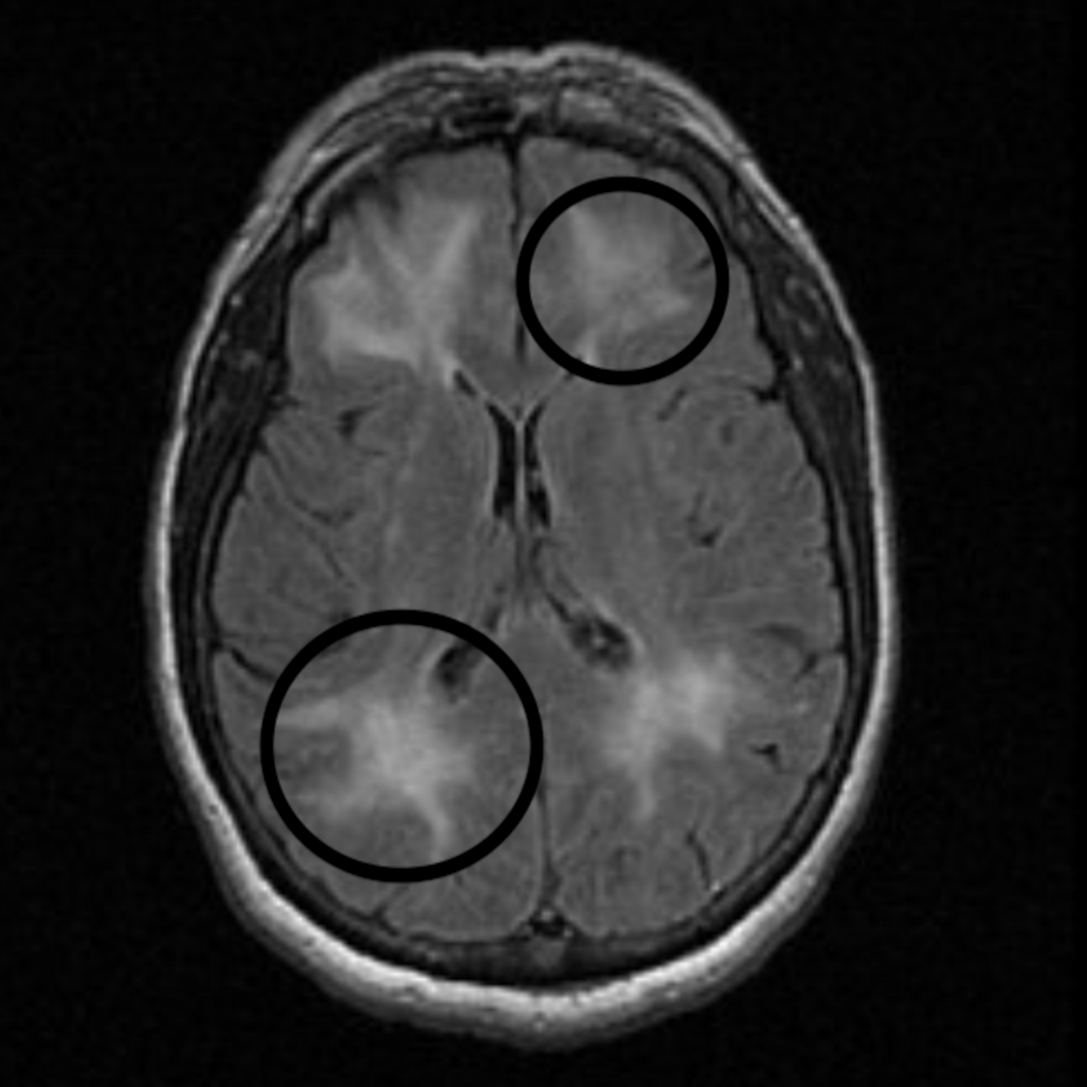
Magnetic resonance imaging (MRI). MRI brain scan showing diffuse white matter changes (circles).
